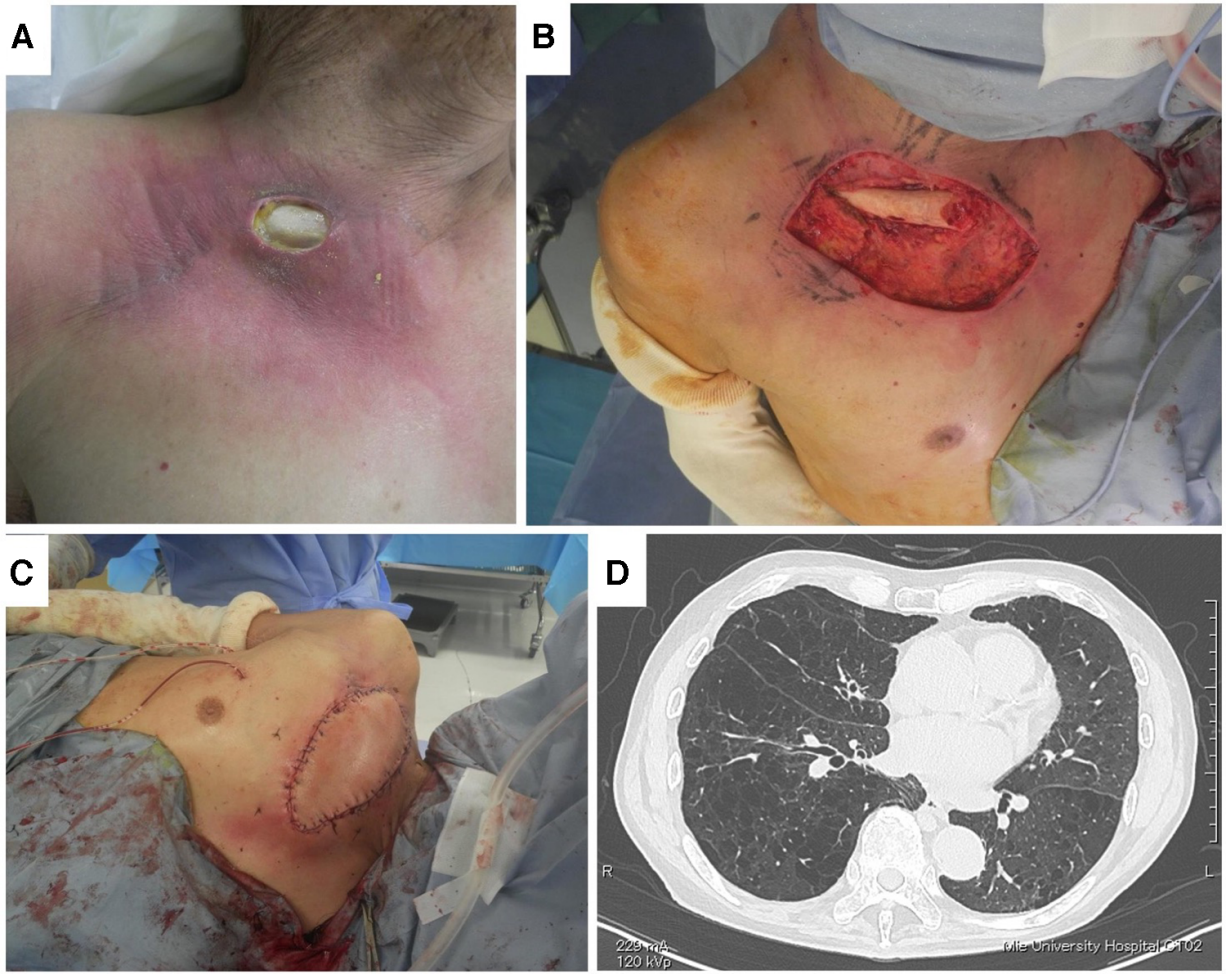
Patient No. 2. Fistula (A), debridement of infected and irradiated area (B), coverage by right LD (C), and lung CT (D).
